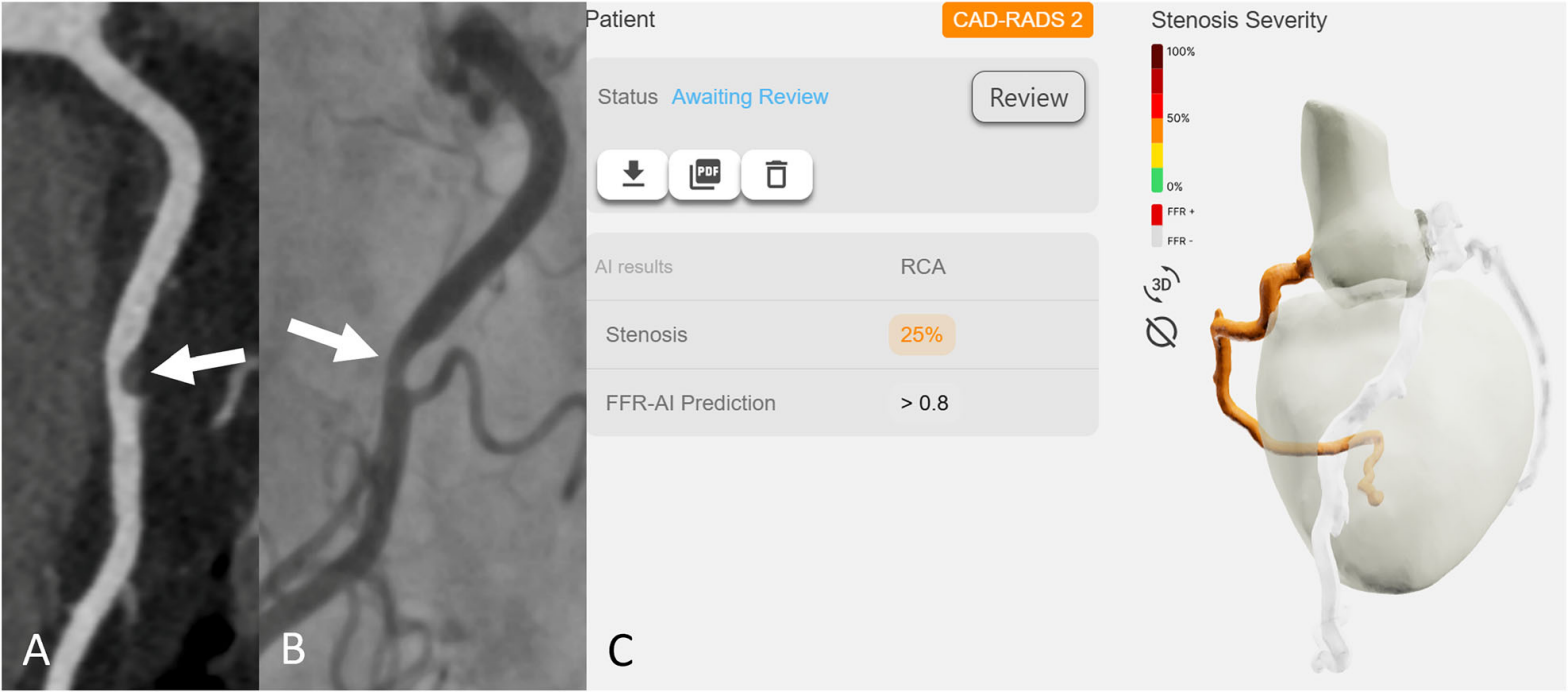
True-negative case. A representative case of a 60-year-old female patient with a recent onset of stable chest pain. Curved multiplanar reconstruction coronary computed tomography angiography A shows a soft plaque at the middle portion of the right coronary artery with obstructive stenosis (white arrow). Invasive coronary angiography B confirms a 26–50% stenosis (white arrow). The Fractional Flow Reserve measurement was 0.94, which is above the 0.8 threshold. Instantaneous wave-Free Ratio measurement was 0.95, which is above the 0.89 threshold. The CT-FFR_AI_ prediction was > 0.8 (C)
